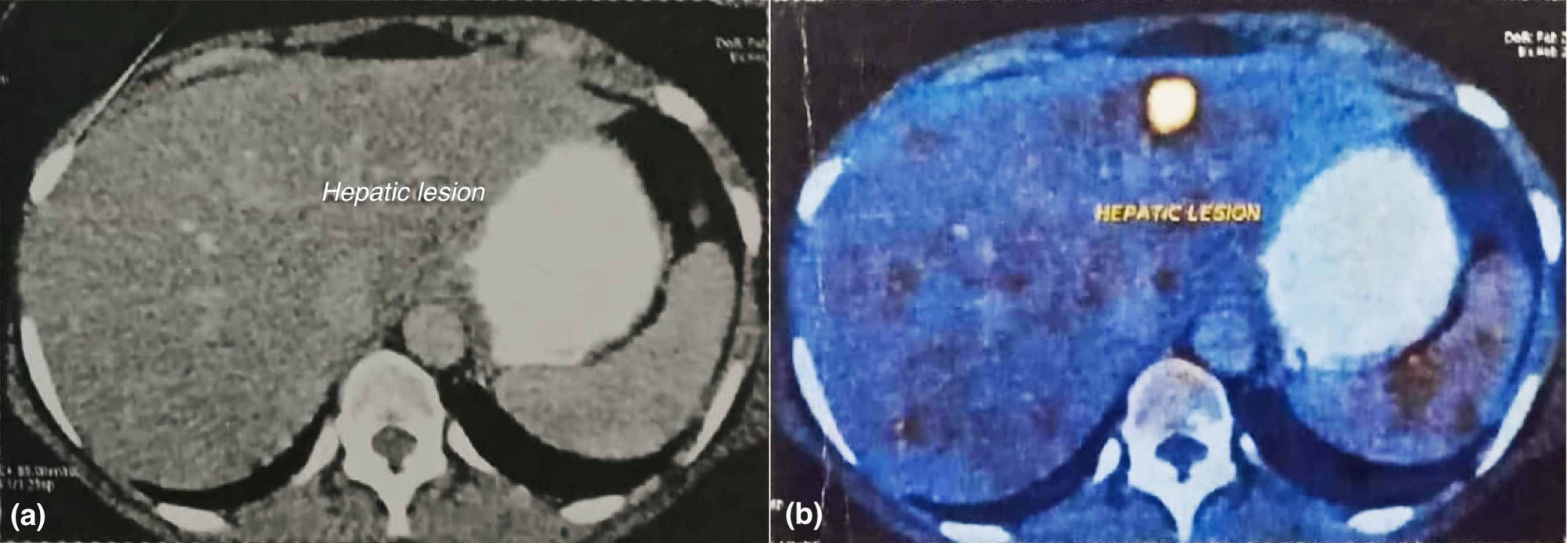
(a) Contrast-enhanced CT abdomen image shows solitary hepatic metastasis. (b) Corresponding fused PET-CT image shows a metabolically active hypodense lesion of size 16 x 14 mm in segment II/III of the left lobe of the liver (standardized uptake value [SUV] Max = 5.5). PET-CT, positron emission tomography-computed tomography.
